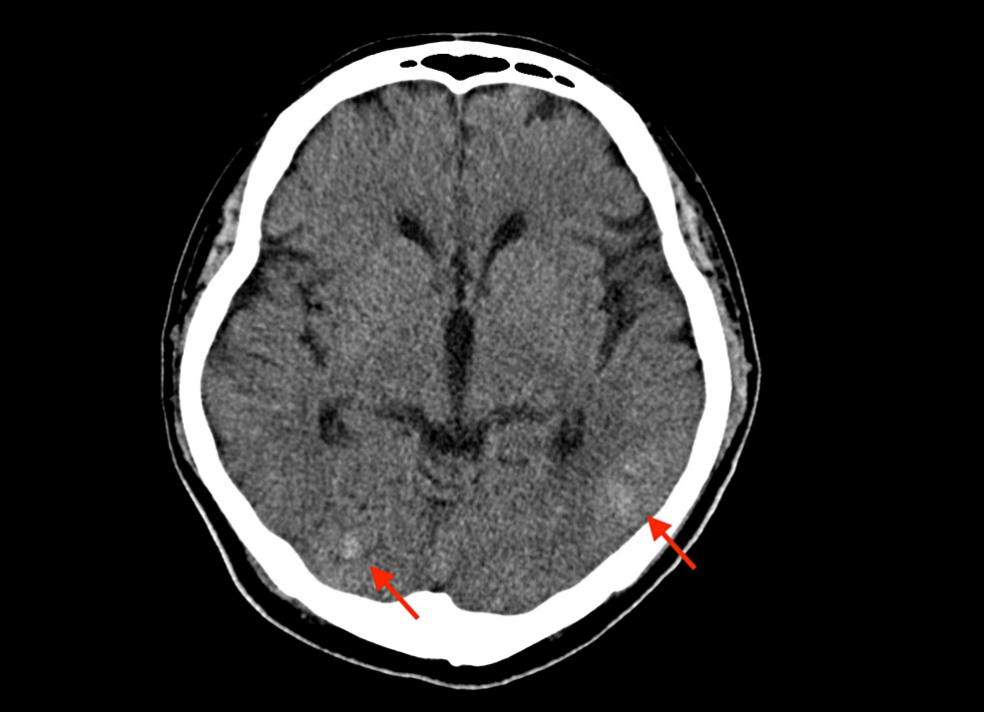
Brain CT showing multiple septic emboli involving bilateral cerebrum (arrows) CT: computed tomography
